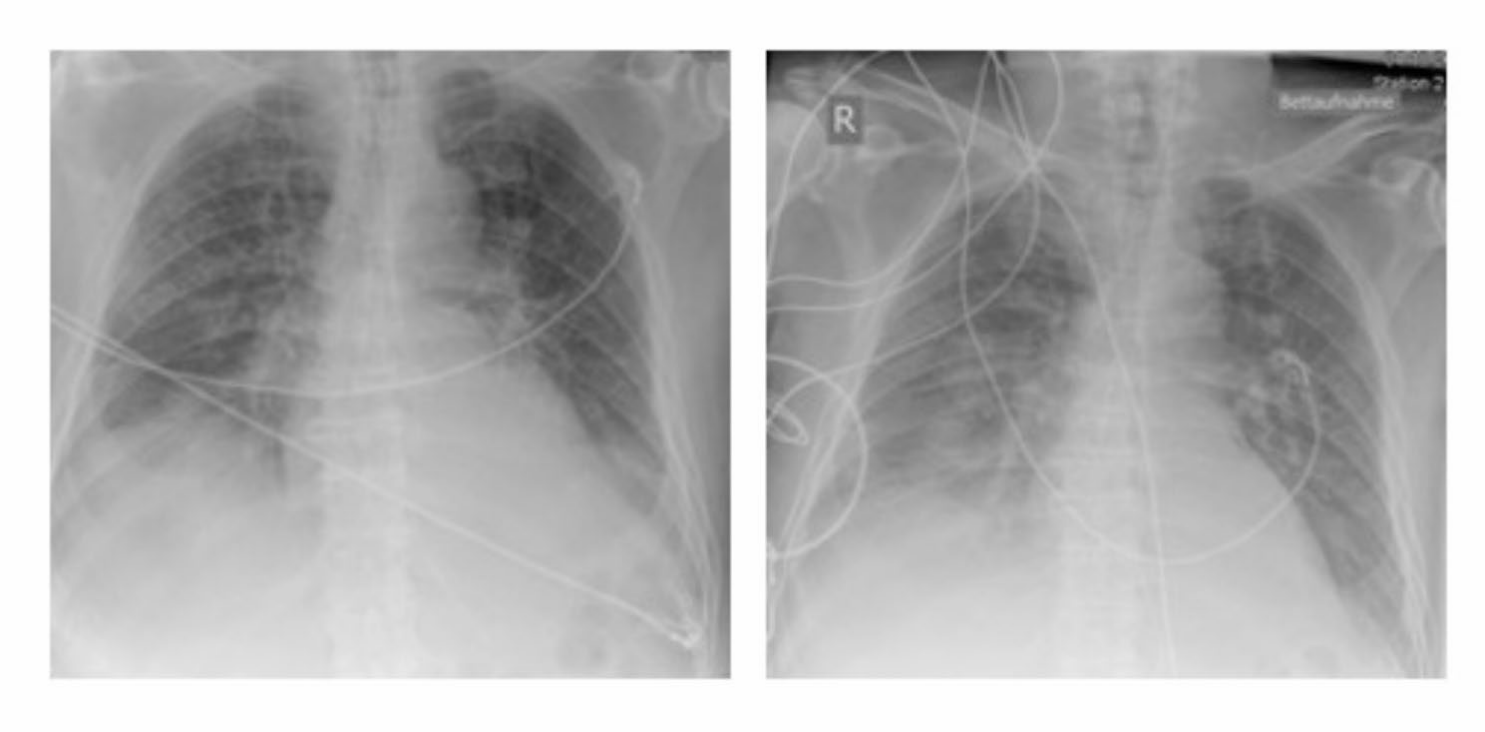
Chest x-rays during ICU stay Left: day of admission to burn unit (1 day after trauma), small dorso-basal effusions on both sides. Patchy increase in markings in the right upper field. Right: day 10 after trauma, clinically deteriorating pneumonia, Small dorsal pleural effusion on the right, with suspected infiltrates on the right basal side.
